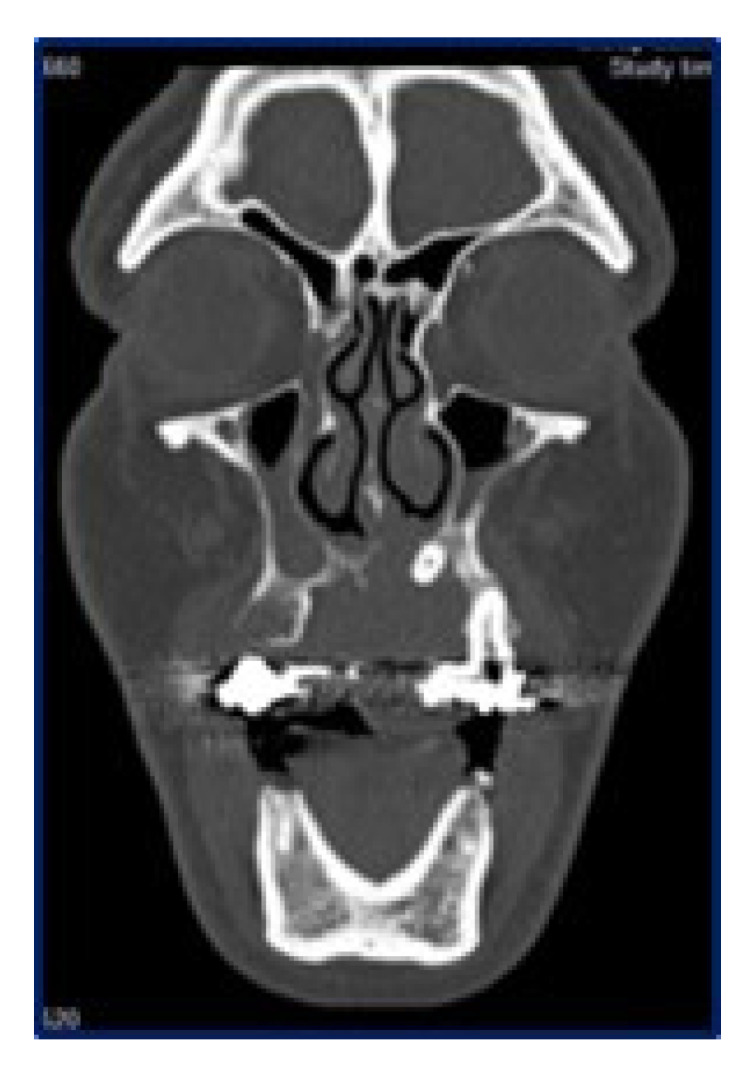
Sagittal computed tomography showing the extension of the lesion, with erosion of the hard palate and involvement of the maxillary sinus up to the orbital base.
